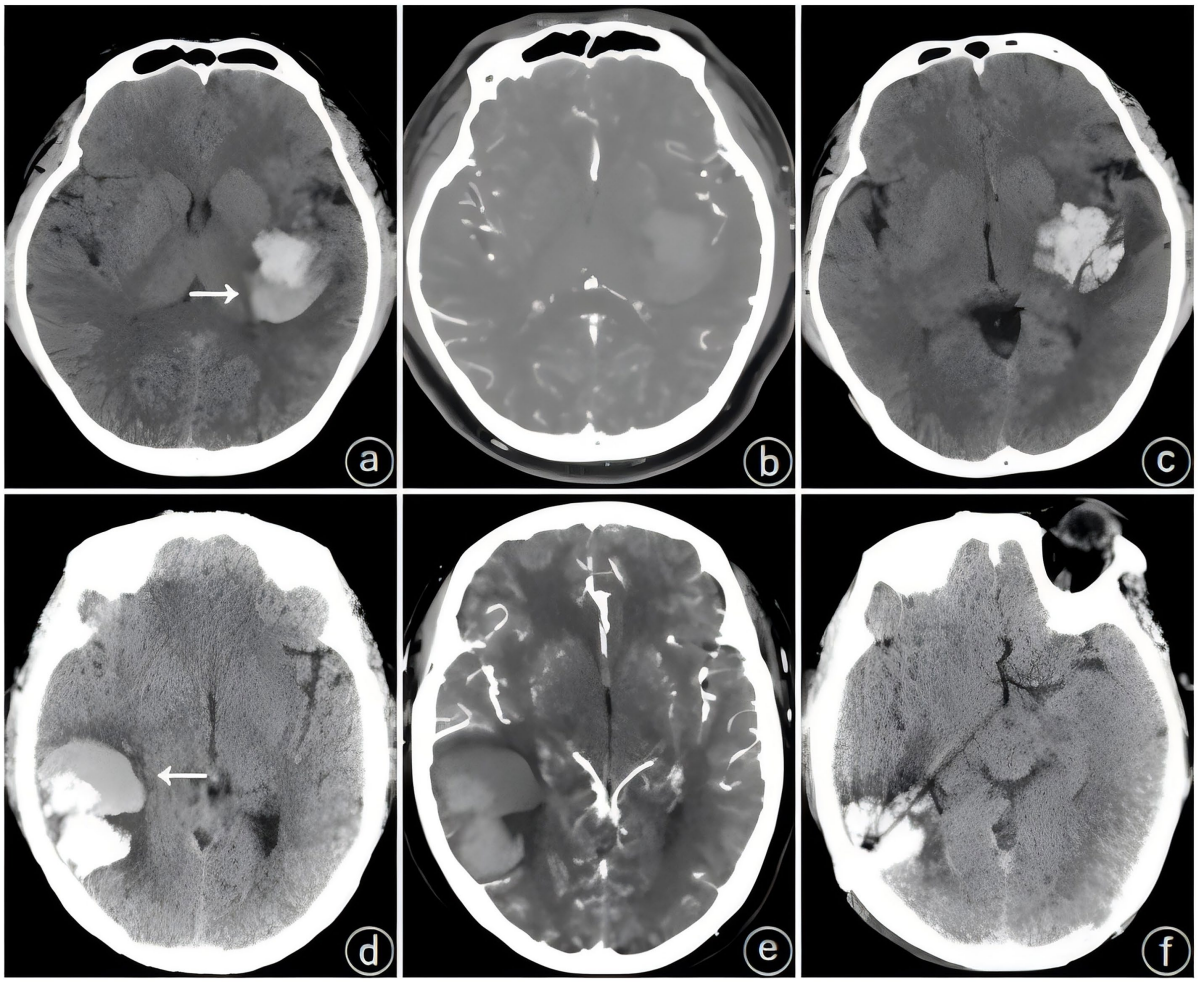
Typical cases of a single blend sign (white arrow). (a–c) Patient: male, 35 years old, (a) initial hematoma 28 mL, (b) no spot sign on computed tomographic angiography (CTA) within 24 h, (c) postoperative review of hematoma expansion (HE). (d–f) Patient: female, 49 years old, (d) initial hematoma 30 mL, (e) no spot sign on computed tomographic angiography (CTA) within 24 h, (f) postoperative review of no hematoma expansion (HE).
